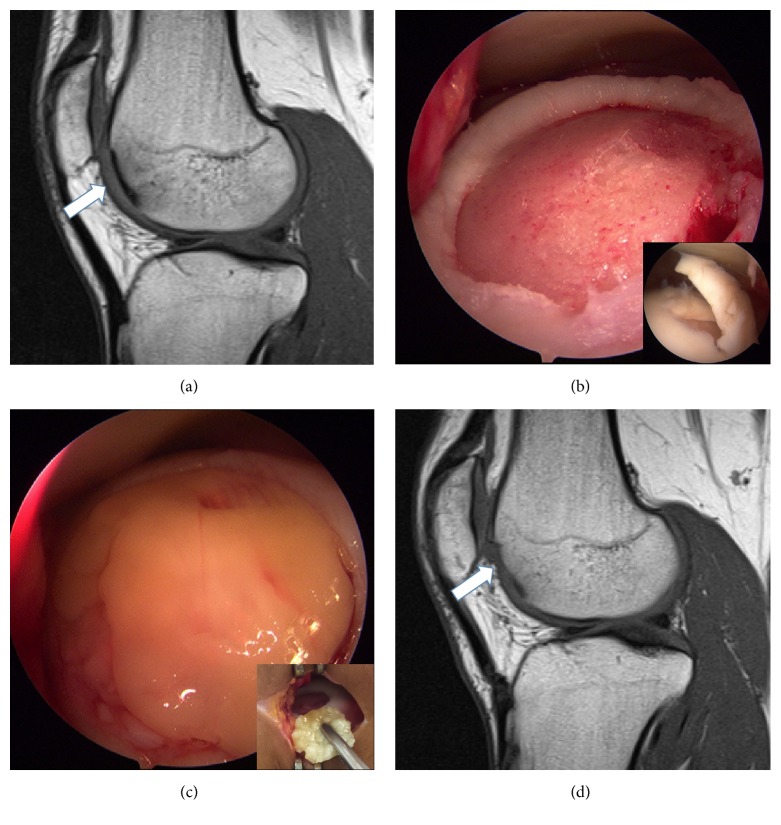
Composition of figures demonstrating the initial injury, surgical treatment, and postoperative MRI 6 months after surgery. (a) Preoperative sagittal T1-sequence MRI demonstrating an osteochondral injury (white arrow). (b) Intraoperative picture demonstrating the debrided defect in the lateral femoral condyle. The small picture shows the injury seen during the diagnostic arthroscopy. (c) Intraoperative picture demonstrating the filled defect after the autologous matrix had been applied. (d) Postoperative sagittal T1-sequence MRI demonstrating adequate filling and incorporation of the matrix in the lateral femoral condyle and absence of subchondral oedema (white arrow).
